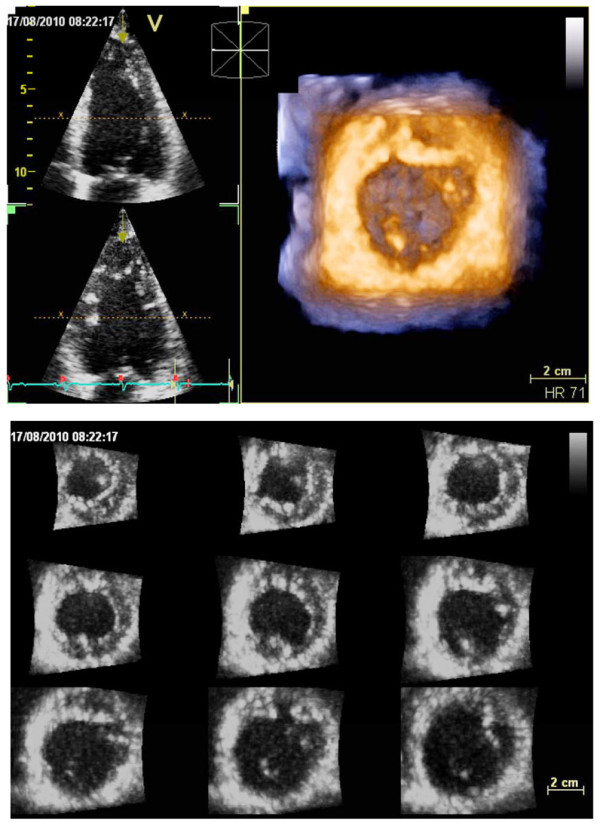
Real time 3-D echocardiography for quantitation of the left ventricle. Care was taken to encompass the entire LV cavity in the data set by checking LV views from 2-D multiplane display and 3-D LV transversal plane (upper panel). After the 3-D acquisition, 9-slice display mode was used to ensure optimal imaging of the entire LV endocardium at each short-axis level and lack of stitching artifacts (lower panel).
